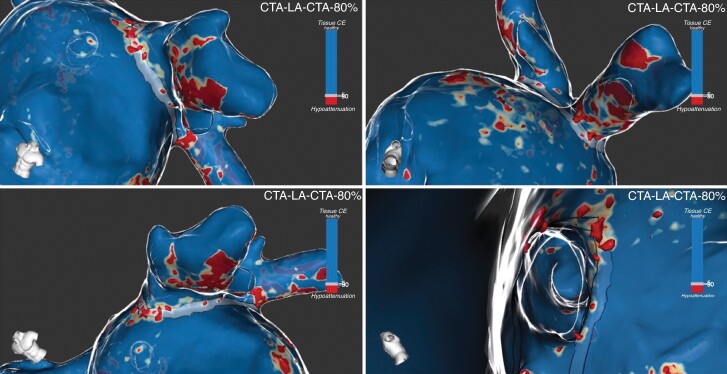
PVI line analysis. MDCT-derived 3D inFAT maps of the LA from different perspectives. A 6 mm wide band corresponding to PVI line trajectory was traced. Top-left panel: lateral–cranial view of the left PVI line; top-right panel: lateral–caudal view of the left PVI line; bottom-left panel: lateral–cranial view of the left PVI line; bottom-right panel: internal medial-to-lateral view of the left PVI line. MDCT, multidetector computed tomography; inFAT, intramyocardial fat; LA, left atrial; PVI, pulmonary vein isolation.
